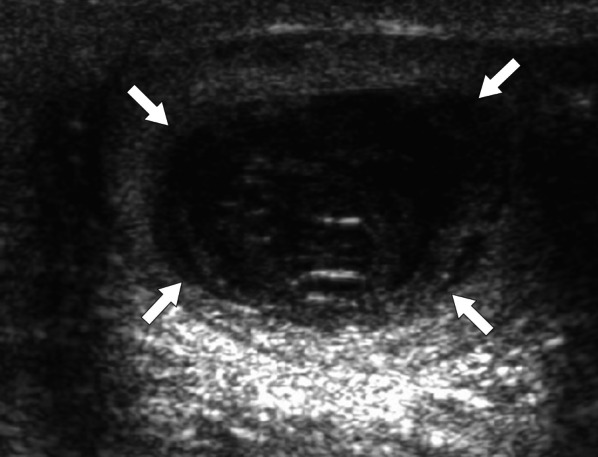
Testicular vasculitis. A 37-year-old male with known Churg-Strauss disease now presenting with new testicular mass. The patient was afebrile and did not report any pain. Ultrasound image demonstrates focal well-defined hypoechoic, centrally cystic mass, almost completely replacing the left testis (arrows). Orchiectomy revealed necrotizing vasculitis with no malignant cells
